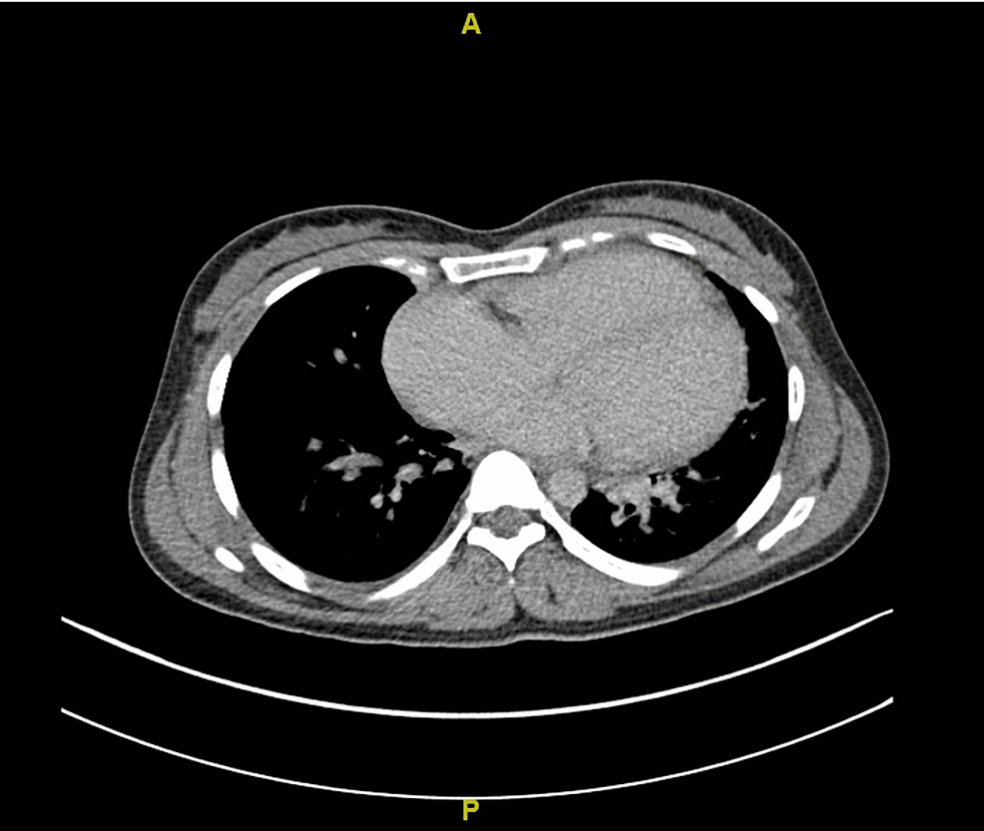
Contrast CT chest showing four-chamber dilatation of the heart with no pulmonary embolism
